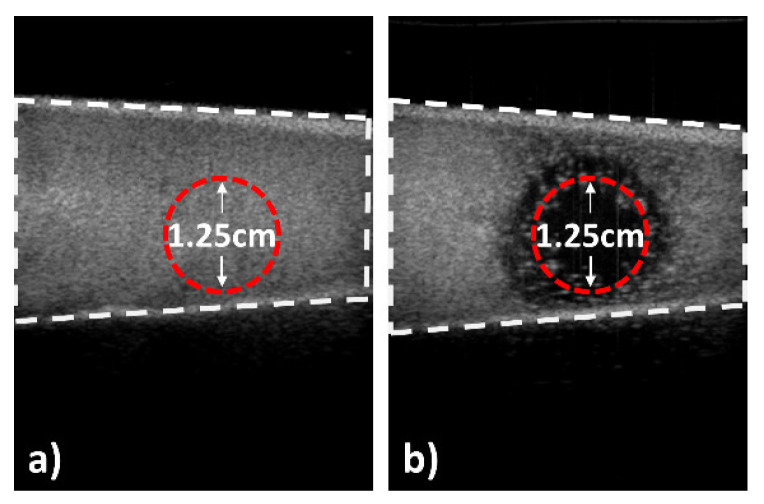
Bubble deletion in ultrasound imaging. An ultrasound system (PICO, Medison, Republic of Korea) with 5–9 MHz linear probe was used for observation. The frame rate was 20 frame/s, and the mechanical index (MI) was 0.9. The mixed solution of degassed water and echogenic liposome with a ratio of 100:1 was prepared and placed inside of the latex chamber. Ultrasound images before and after sonication were obtained. Ultrasound parameters were 1 MPa peak positive pressure with a 1% duty factor, 3.05 × 10^−1^ W/cm^2^ I_SPTA_. (a) is an ultrasound image before sonication, and (b) is an image right after starting sonication. The time between the two images was less than 100 ms. The affected area became dark immediately due to the destruction of echogenic liposomes. The dotted line indicates the active area of transducer which has similar size of darken area in the image. This indicates that the chosen parameters can be used for the effective disruption of echogenic liposomes over the sonicated area with a size that is close to the transducer, even though the focus size of the 6 dB transducer was only approximately 4 mm on the focal plane.
